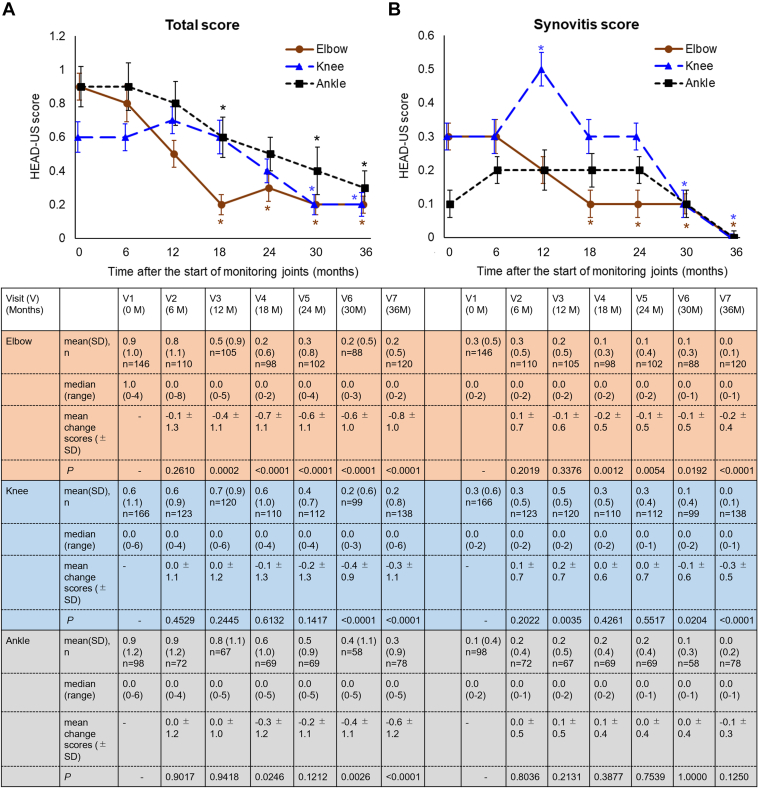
Improvement in the HEAD-US score obtained by MSKUS joint monitoring over 3 years. The HEAD-US scores of the right and left joints (elbow, knee, and ankle) were collected, and the higher score of each participant was used to calculate descriptive statistics. (A) HEAD-US total score combining HEAD-US synovitis, cartilage, and bone scores for the elbow, knee, and ankle. (B) HEAD-US synovitis score. The table header corresponds to time-point labels for graphs. Error bars represent SEs. ∗ represents significant differences with *P* < .05, as determined by the Wilcoxon signed-rank test. HEAD-US, hemophilia early arthropathy detection with ultrasound; MSKUS, musculoskeletal ultrasound; *n*, number of participants with hemophilia.
